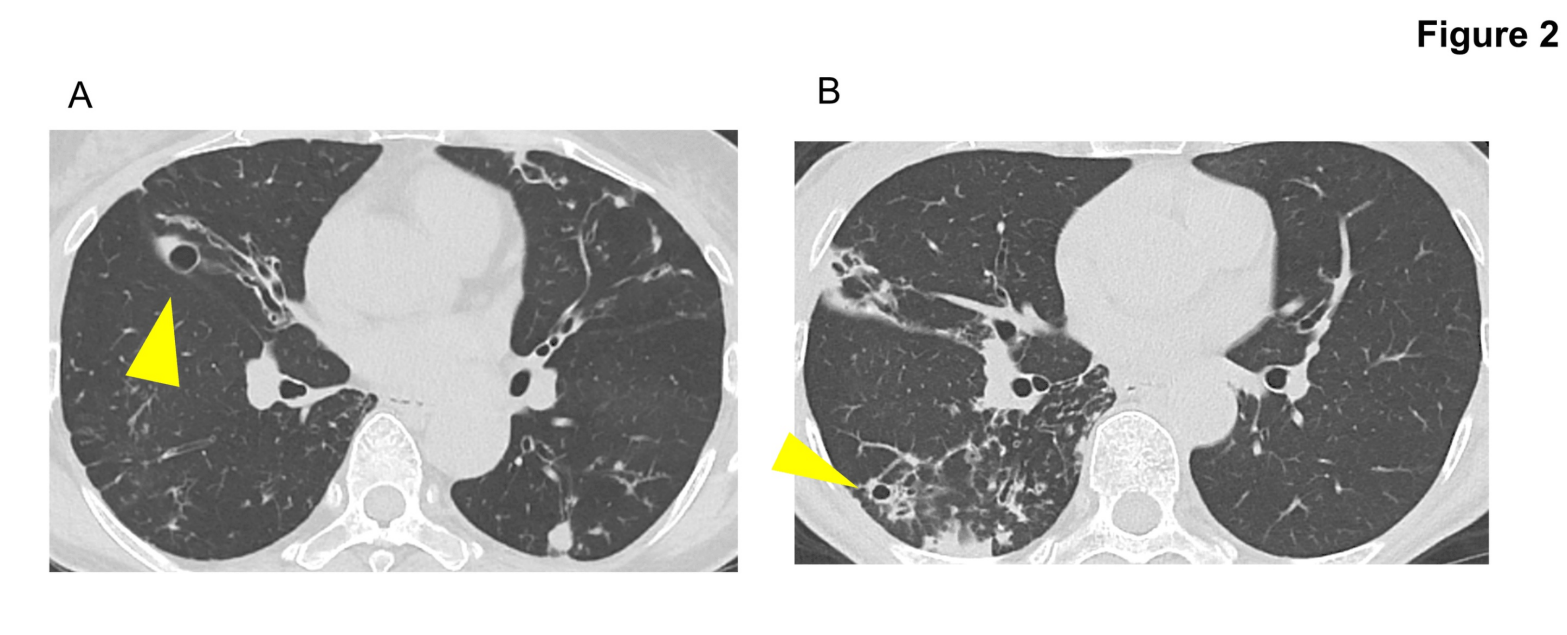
Chest CT before the administration of STFX and ALIS in cases 3 (non-responder) and 4 (non-responder) of CAM-resistant pulmonary MAC disease (A) Chest CT before STFX+ALIS in case 3. The arrow shows the cavity. (B) Chest CT before STFX+ALIS in case 4. The arrow shows the cavity. Abbreviations STFX: sitafloxacin, ALIS: amikacin liposome inhalation suspension, CAM: clarithromycin, MAC: *Mycobacterium avium* complex
